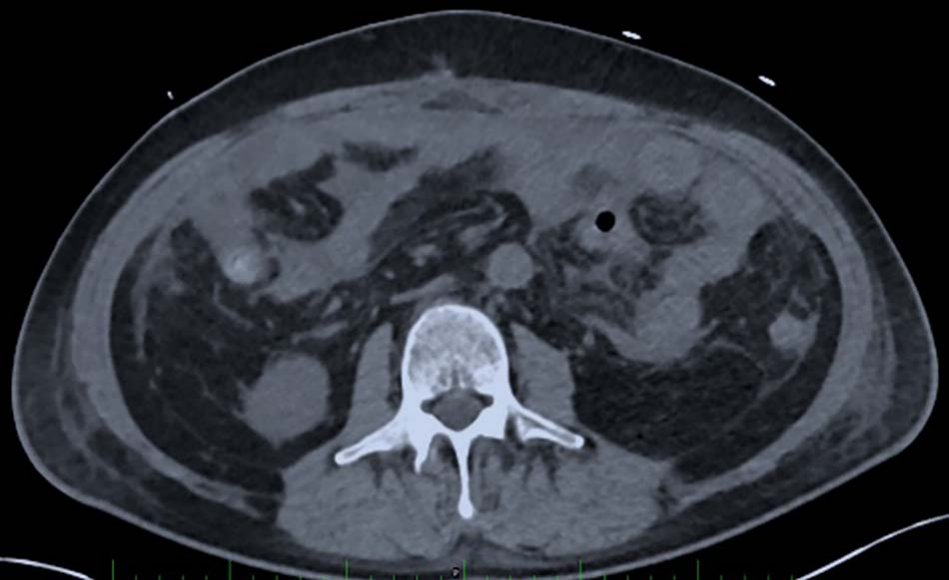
Post-operative Day 20. Abdominal CT-scan with free intraperitoneal fluid, without sign of ileus, perforation or anastomosis release.
